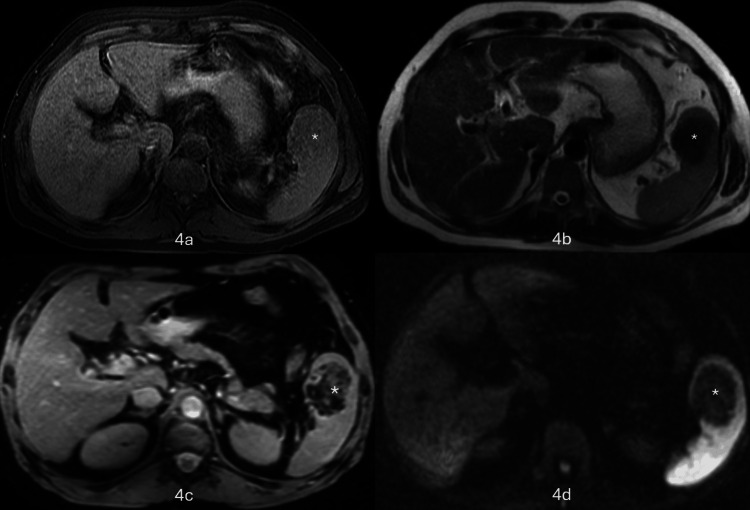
MRI of the abdomen MRI showing a T1-isointense lesion (star) with respect to the spleen (4a) and a T2-heterogeneously hypointense lesion (4b). Susceptibility artefact on GRE sequence (star) is suggestive of hemosiderin deposition within the lesion (4c). There is no evidence of diffusion restriction (star) on diffusion-weighted imaging (DWI) sequence (4d).
